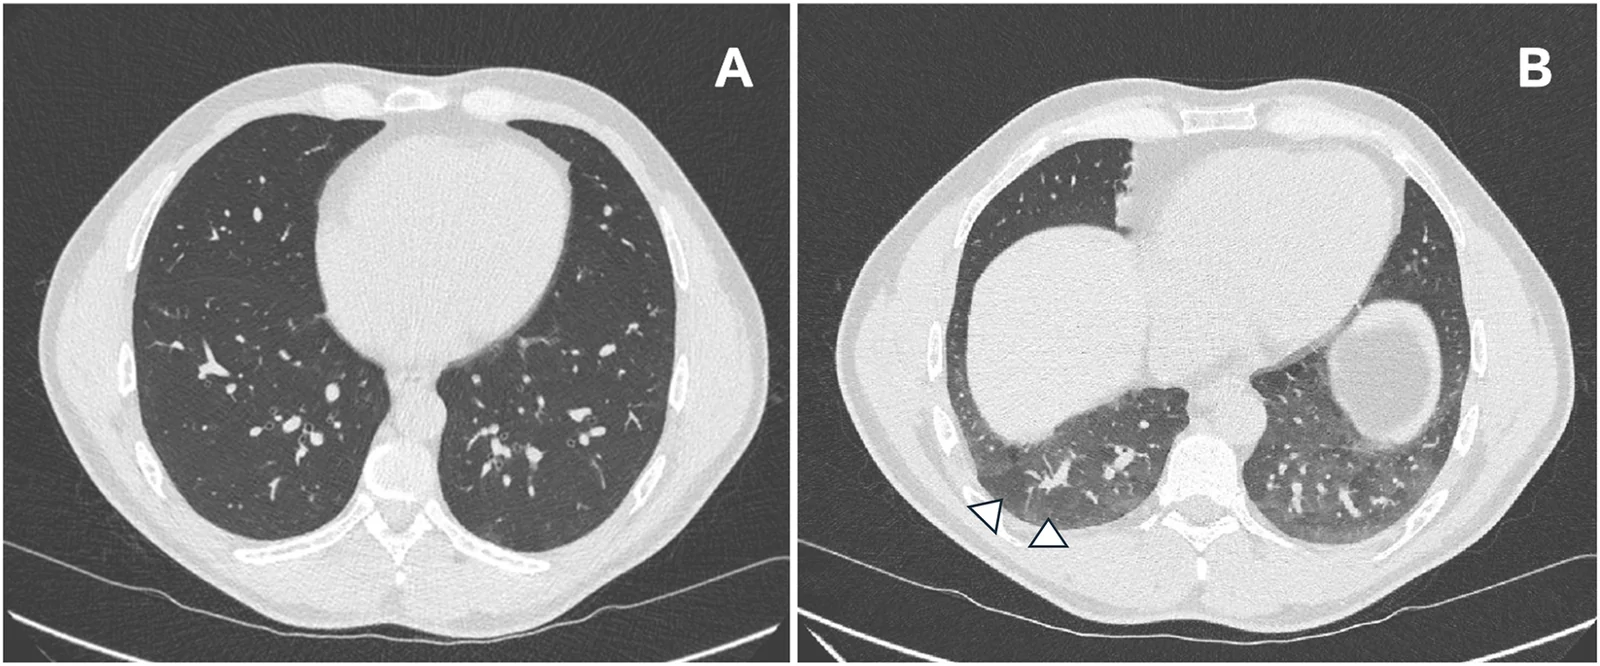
Axial images of the thorax computed tomography 1 month after the exposition, on inspiratory phase (A) and expiratory phase (B). A mosaic pattern, consistent with small airway disease, of the lower lobes is present on the expiratory acquisition (differential attenuation, arrowheads).
